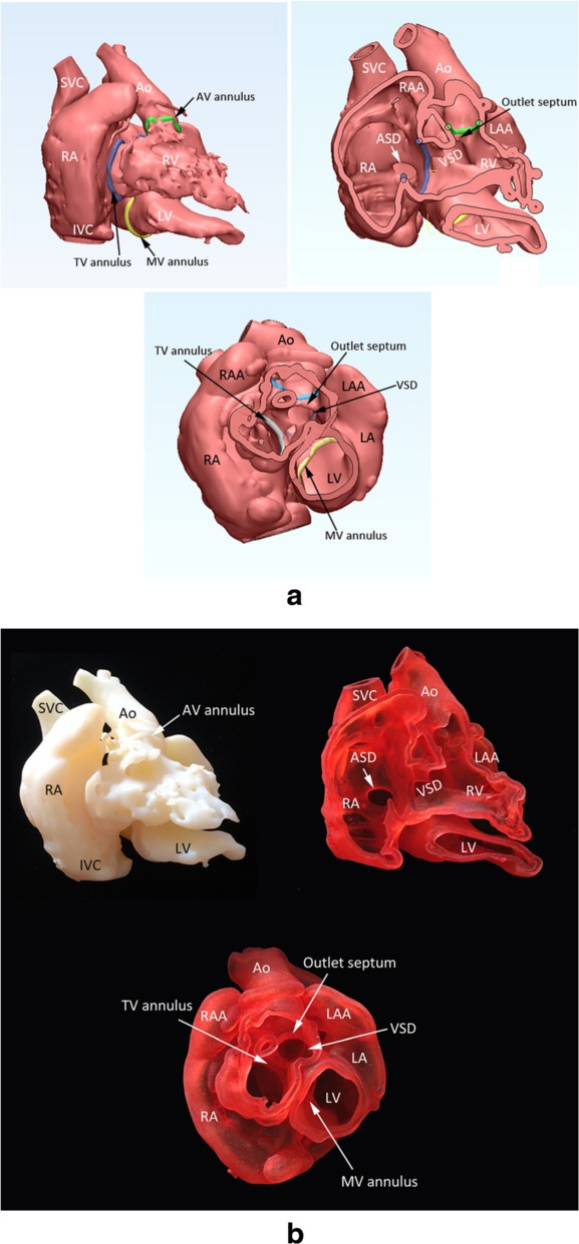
Screen-display of the STL files (a) and photographs of the corresponding models (b) in a case with so-called twisted or criss-cross heart with transposition of the great arteries and a ventricular septal defect (VSD). Cast model (top left), wall model after removal of the anterior free wall of the right atrium and right and left ventricles (top right), and wall model with the apical two thirds of the ventricles removed (bottom) are shown. The cardiac valve annuli are marked by the spline curves that were graphically designed as shown in Fig. 2B. Ao, aorta; ASD, atrial septal defect; AV, aortic valve; IVC, inferior vena cava; LA, left atrium; LAA, left atrial appendage; LV, left ventricle; MV, mitral valve; SVC, superior vena cava; TV, tricuspid valve
